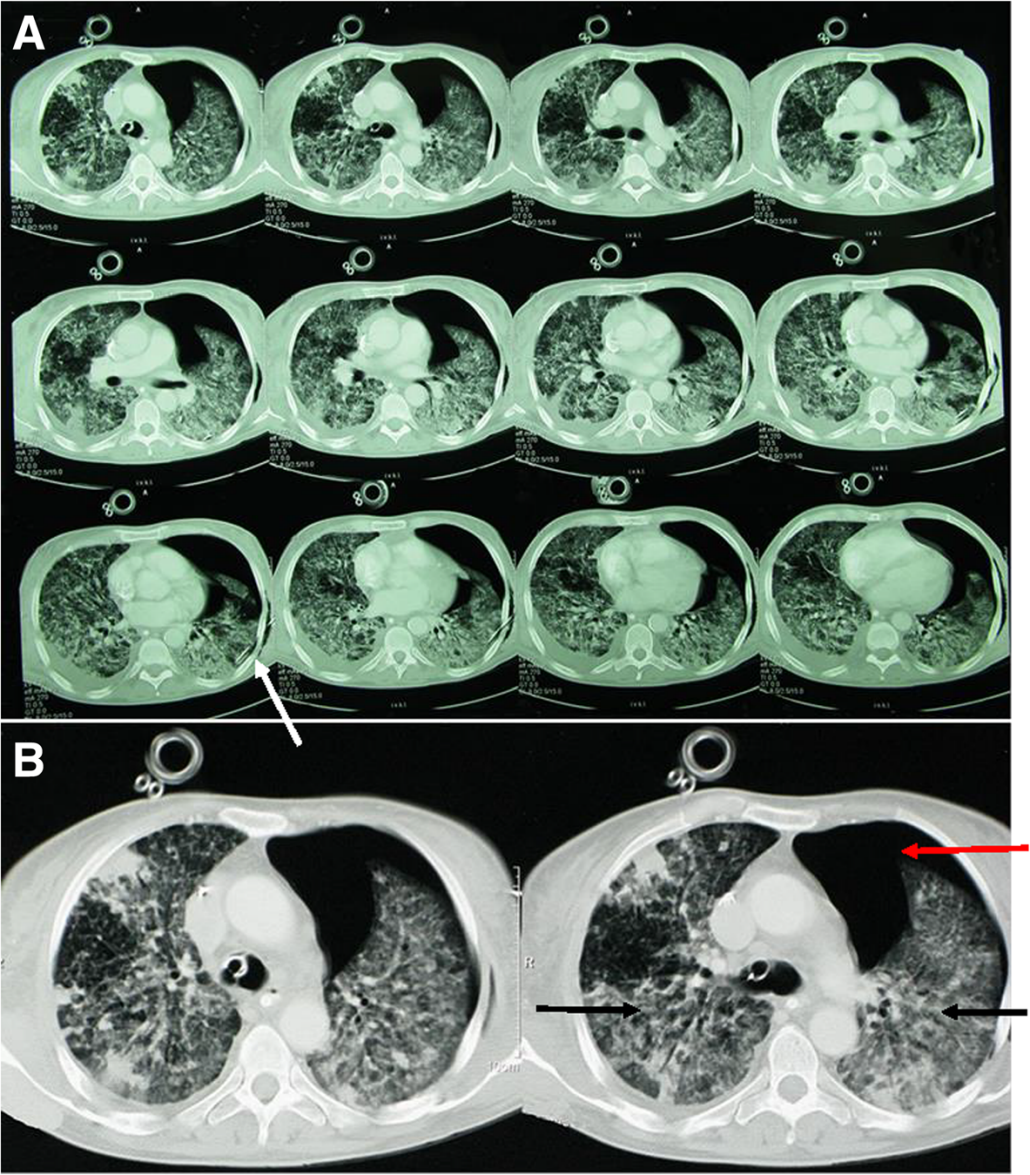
Computer tomography scans at day 1 of admission. CT scan shows acute respiratory distress syndrome (ARDS), diffuse bilateral infiltrates (black arrows), pneumothorax (red arrow) and chest tube (white arrow) (a), detailed CT scan (b)
